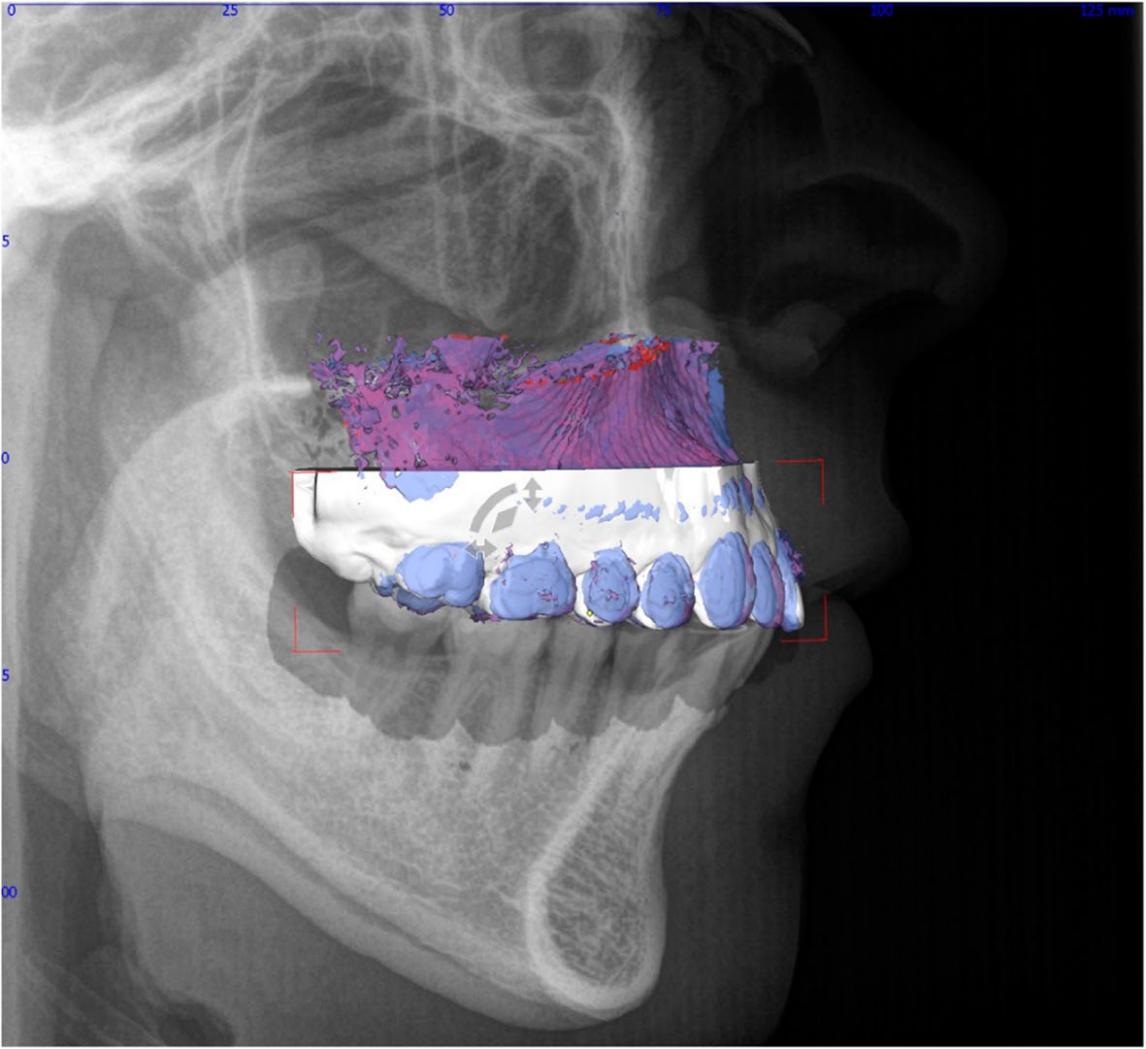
Data set after three-dimensional superimposition of lateral cephalogram, intraoral scan of the maxilla, and CBCT
